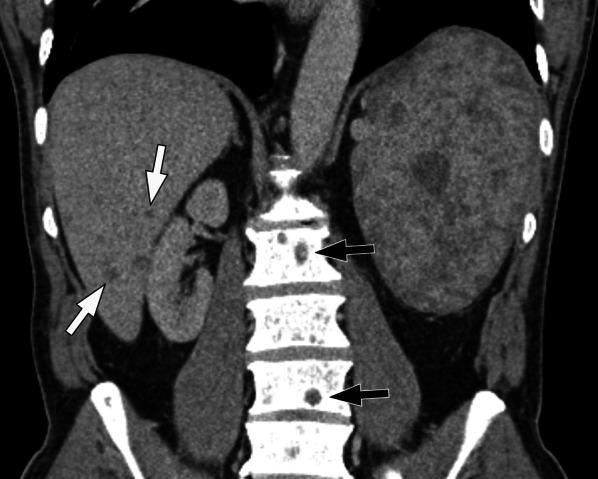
66-year-old man with primary splenic angiosarcoma. Coronal contrast-enhanced CT shows multiple low attenuation lesions of varying sizes within an enlarged spleen. There are multiple low attenuation nodules within the liver (white arrows) and lytic lesions (black arrows) within the vertebral bodies in keeping with liver and skeletal metastases
